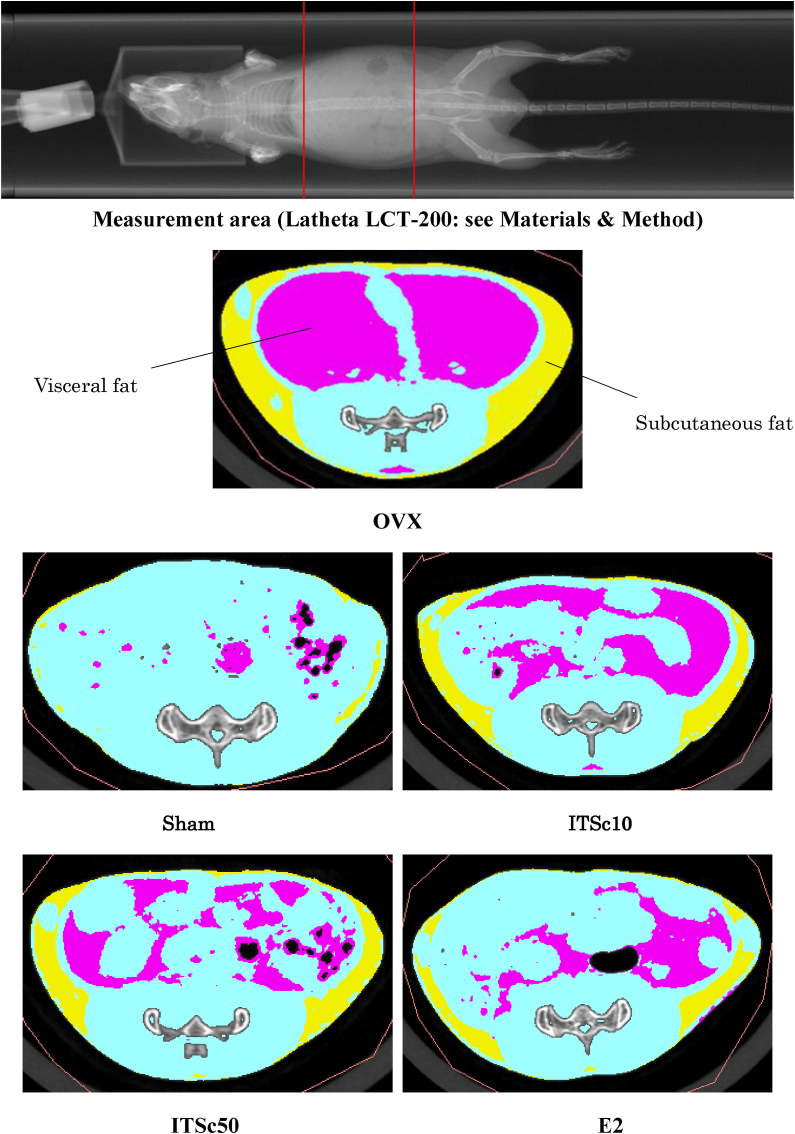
Typical abdomen image in CT imaging.
